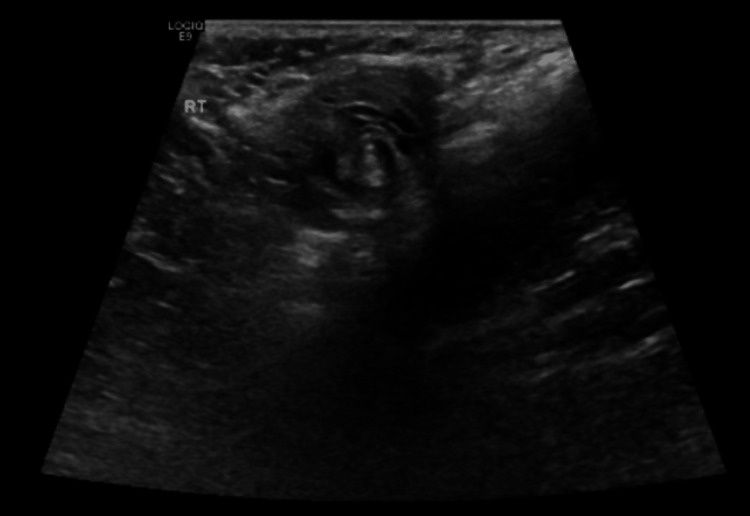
Preoperative scrotal Doppler ultrasonography displaying absent intratesticular arterial flow on the affected side, consistent with testicular torsion.
